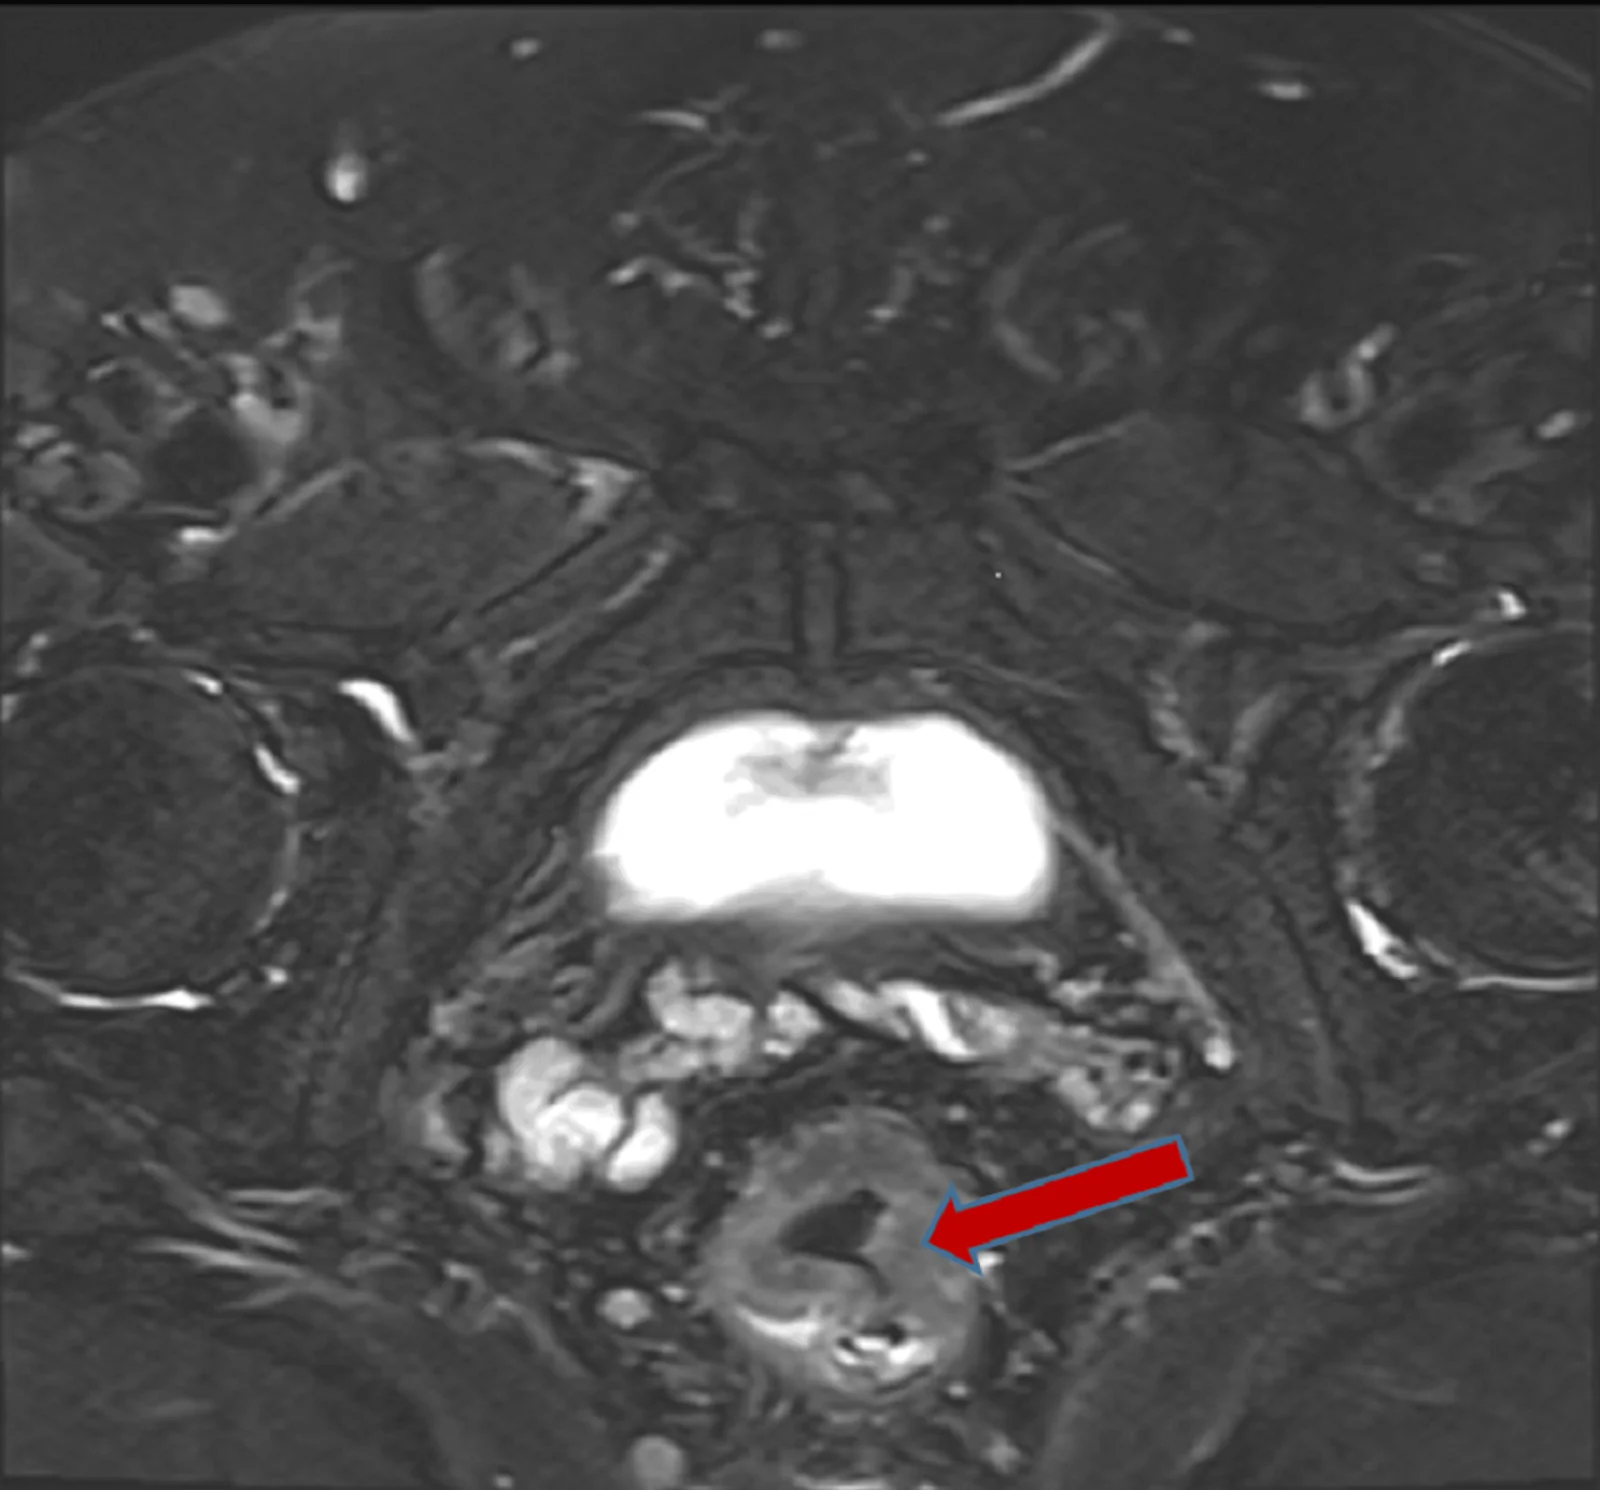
Magnetic resonance imaging of the pelvis showing the rectal tumor before treatment (arrow)
